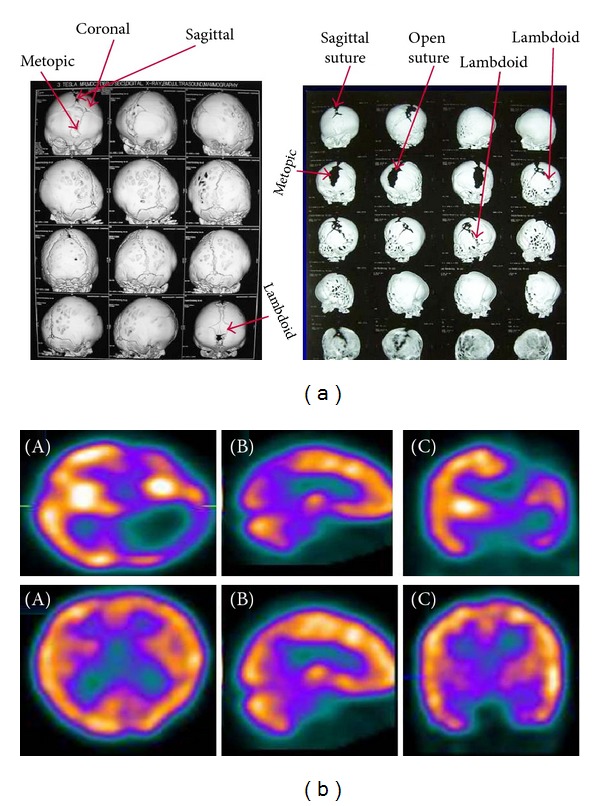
(a) CT volumetric rendering technique (VRT) images showing fusion of left fronto-parieto-temporo-occipital lobe sutures preoperatively (A) and release of all these sutures postoperatively (B). (b) ^99m^Tc-ECD brain SPECT preoperatively showing absent radiotracer uptake involving left fronto-parieto-temporo-occipital lobe suggestive of hypoperfusion, upper panel images axial (A), sagittal (B), and coronal (C), and postoperatively showing increased radiotracer uptake involving left fronto-parieto-temporo-occipital lobe suggestive of improved perfusion, lower panel images axial (A), sagittal (B), and coronal (C).
